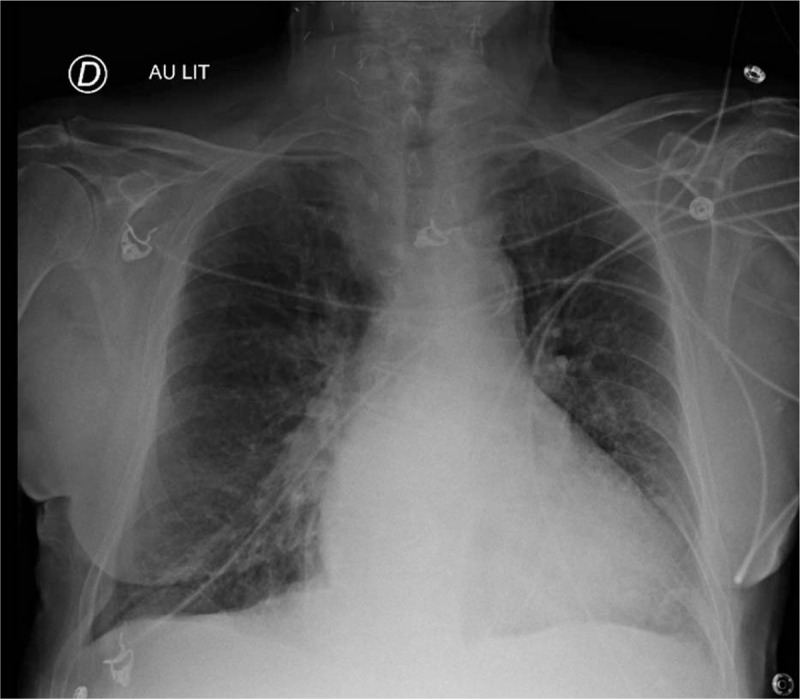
Chest X-ray at admission showing thoracic distension.
